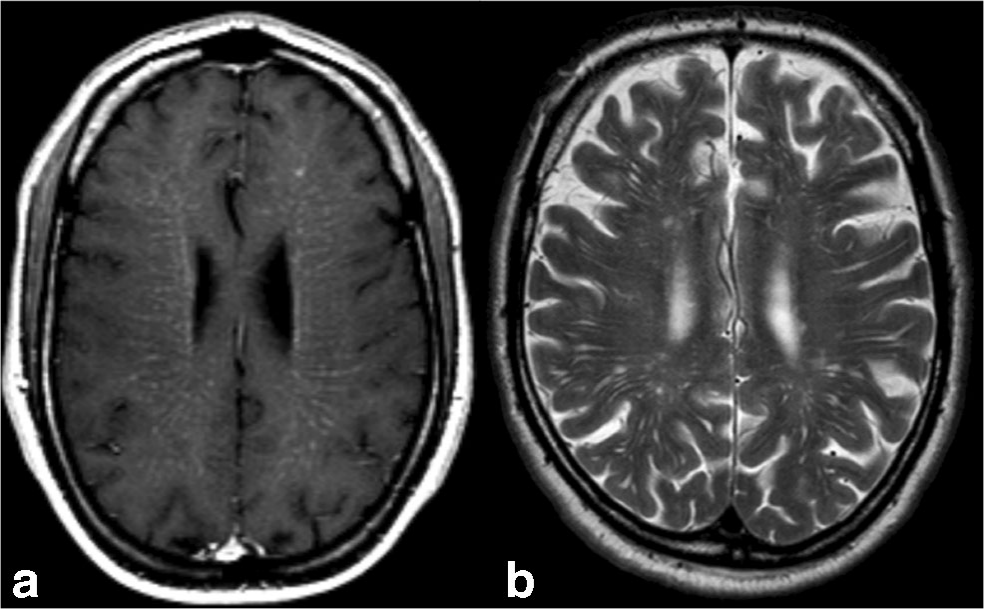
a Axial post-contrast T1-weighted MRI at the level of the corona radiata in a 39-years-old male affected by vitamin B_12_ deficiency. Note the miliary-enhancing nodules in addition to linear enhancement along the pathway of perforating vessels, suggestive for PVS involvements. In this patient, brain abnormalities totally resolved after vitamin B_12_ suppletion therapy. b Note on a T2-weighted MRI in a different and healthy subject the T2 hyperintense prominent PVS identical to the enhancement pattern
